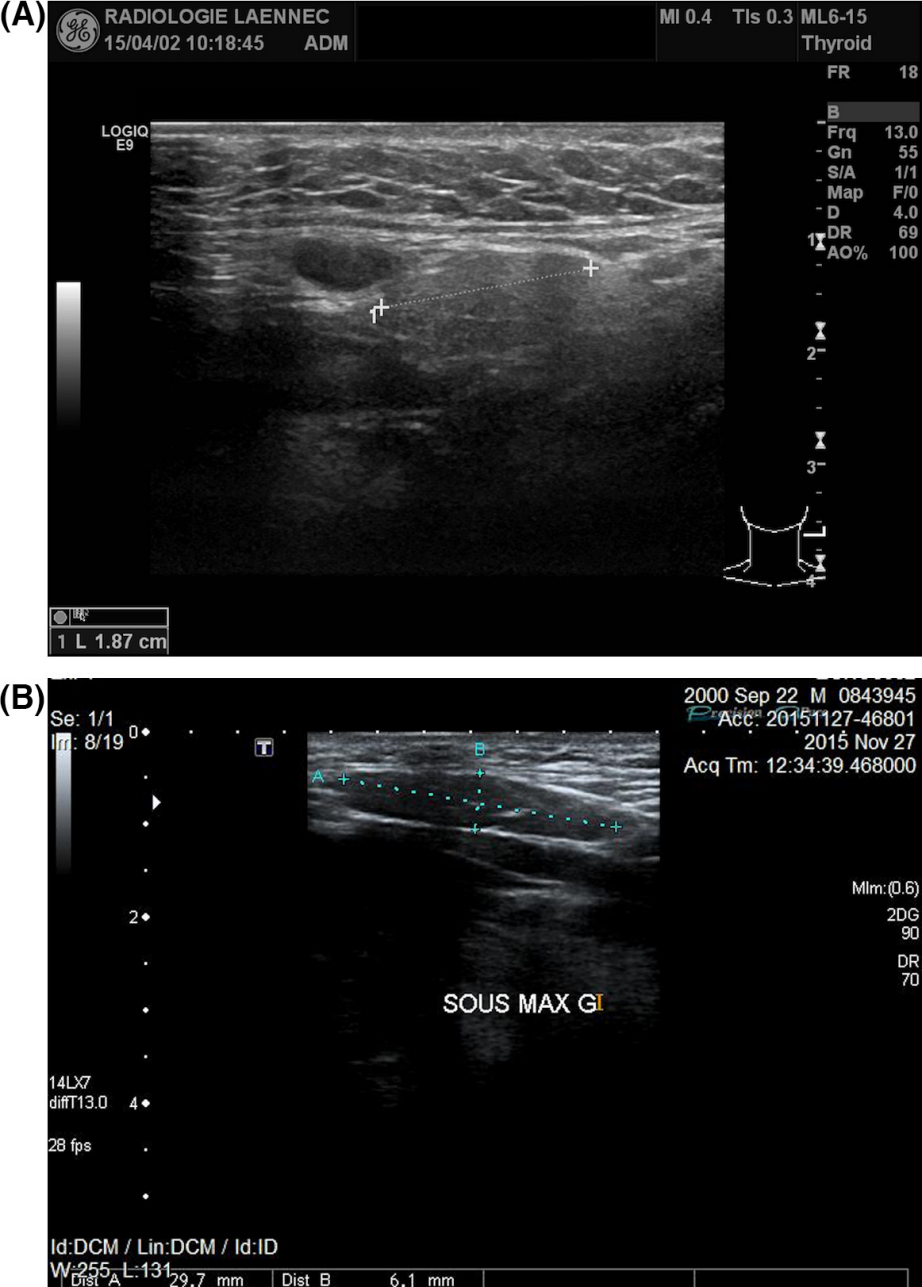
Ultrasonography demonstrating the hypoplastic left submandibular gland in the presented case (A) compared to the ultrasonography of the left submandibular gland in a healthy 16‐year‐old male (B).
